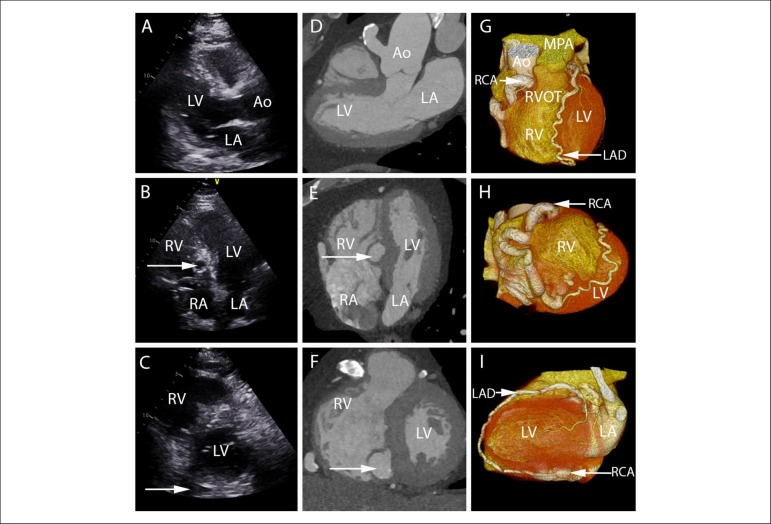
A-C) show the parasternal long axis (1A), apical 4-chamber (1B) and short
